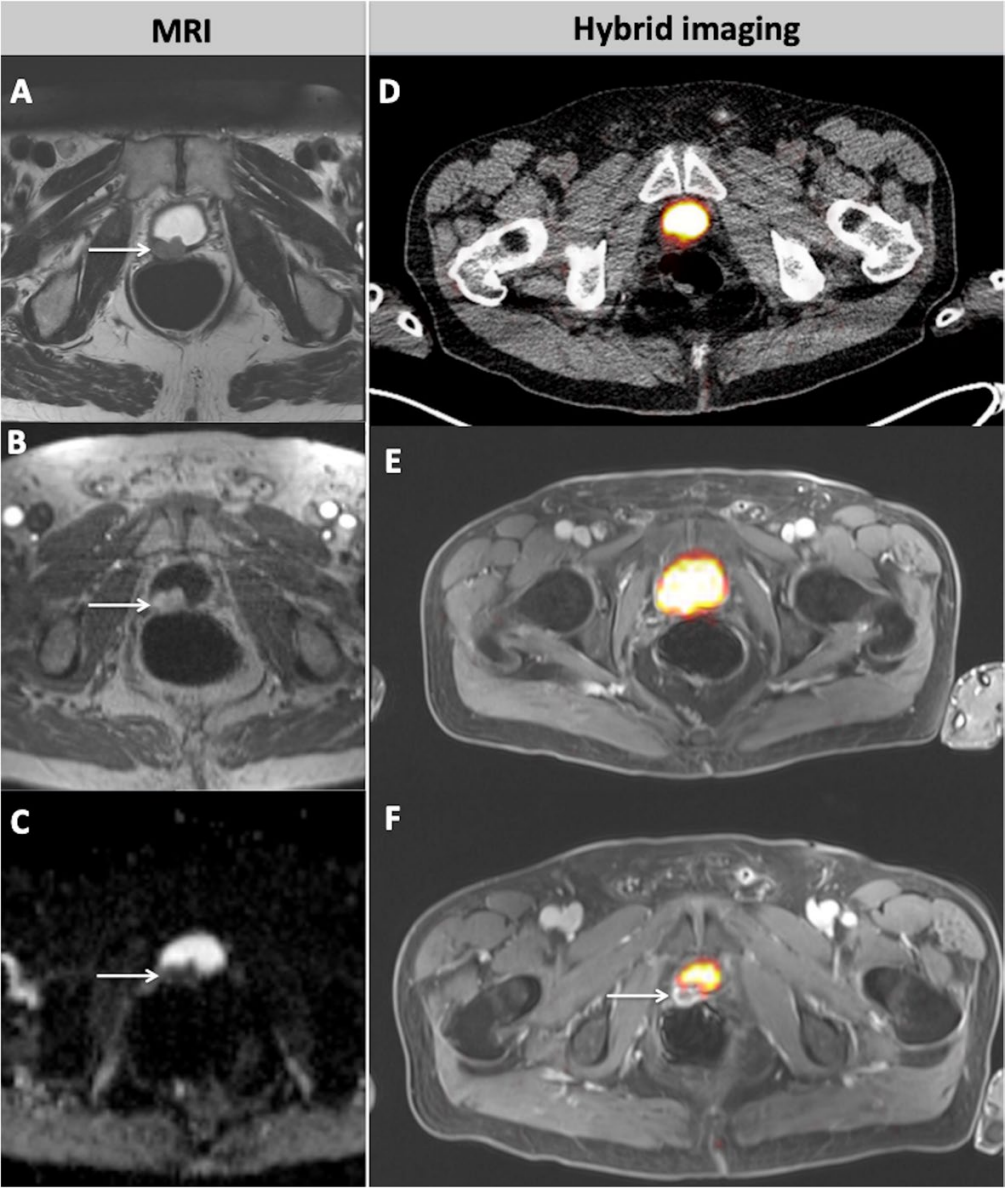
A 66-year old patient referred for imaging due to biochemical failure of PCa after radical prostatectomy and ADT. The patient presented with a PSA level of 1.2 ng/ml. In MRI, a lesion in the prostate bed with hypointense to intermediate T2w signal, suspicious for PCa local recurrence is depicted (A, arrow). The lesion shows early contrast enhancement in the dynamic T1w (B, arrow) and distinct diffusion restriction with signal decrease in ADC map (C, arrow). However, in PET-CT (D) no pathological tracer uptake can be delineated. In PET-MRI, the cranial part of the local recurrence is masked by the physiological [^68^Ga]PSMA-11 accumulation in the urinary bladder (E), while its caudal part can be clearly delineated as a contrast enhancing lesion in the MRI part of the fused image (arrow, F), despite the lack in [^68^Ga]PSMA-11 uptake
